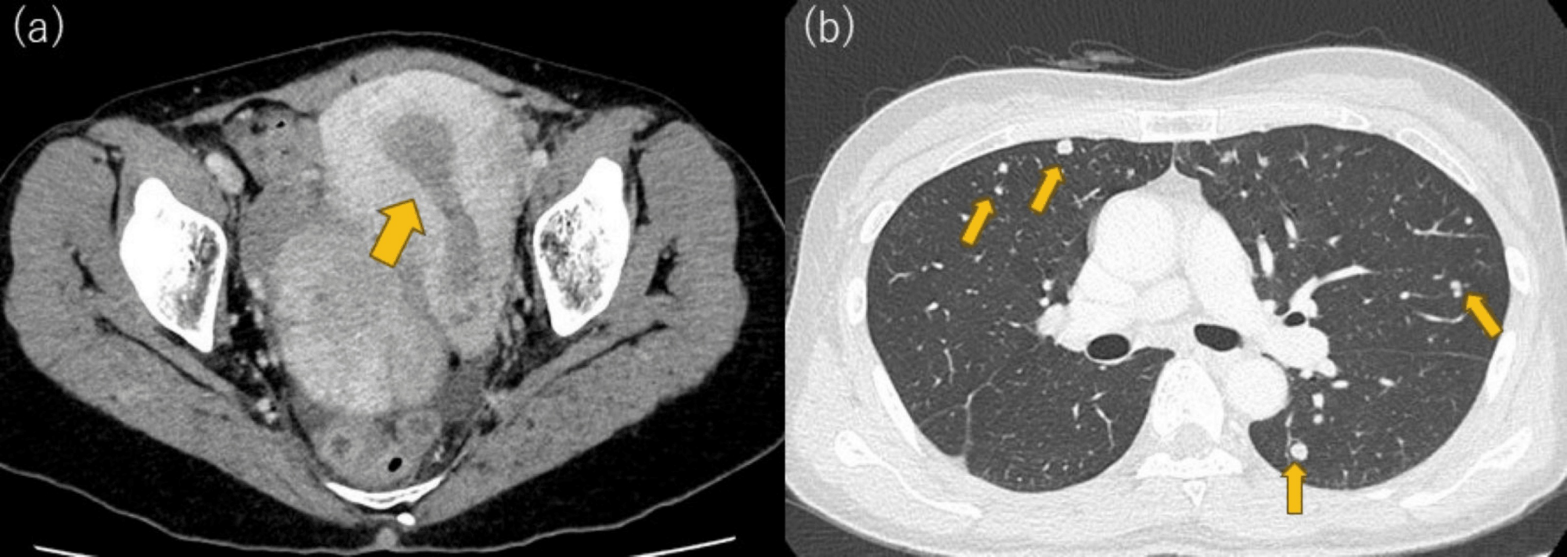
Contrast-enhanced CT showing endometrial thickening (a) and multiple pulmonary nodules consistent with metastases (b) (yellow arrows) CT: computed tomography
